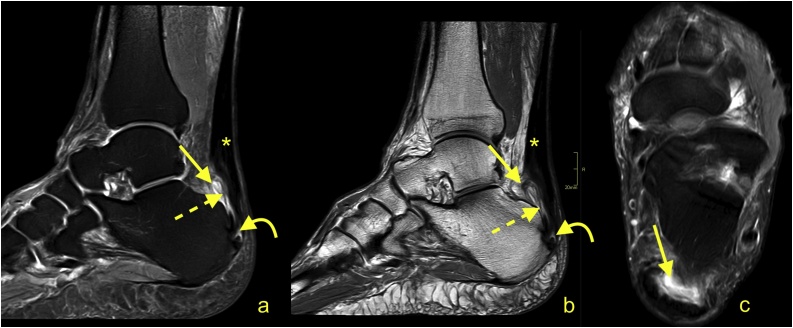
A 26-year-old patient with a suspicion of spondylarthritis presenting with discomfort in the insertion of the Achilles tendon. a and c- T2-weighted with fat suppression; b - T2-weighted. MRI revealed retrocalcaneal bursitis (dashed arrow) and bone marrow edema in the Achilles tendon insertion, however no erosions are present. Thickening of the Achilles tendon in midportion is visible (*), however no alteration in the signal of the Achilles tendon was revealed. A-T2- weighted, b- PD- weighted, c- PD-weighted with fat suppression.
